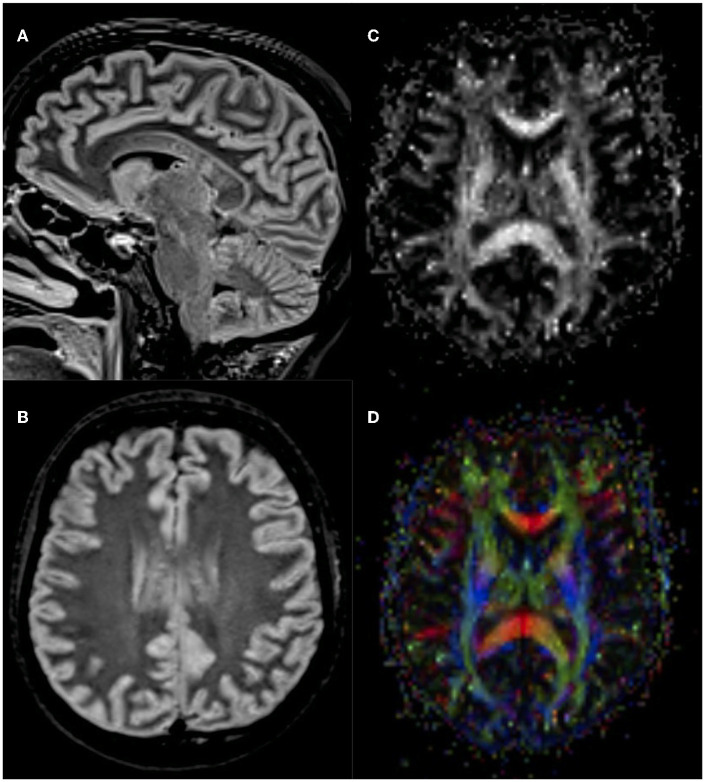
Brain MRI findings. Axial (A) and sagittal (B) 1.5 Tesla T2-weighted fluid-attenuated inversion recovery (FLAIR) showed multiple focal punctate areas of hyperintensities localized in the splenium and isthmus of the corpus callosum, with no contrast enhancement or diffusion restriction. Follow-up 3 Tesla brain MRI revealed no T2-weighted abnormalities (images not shown). Yet, diffusion tensor imaging (DTI) showed fractional anisotropy reduction within the corpus callosum's body and splenium (C, D).
